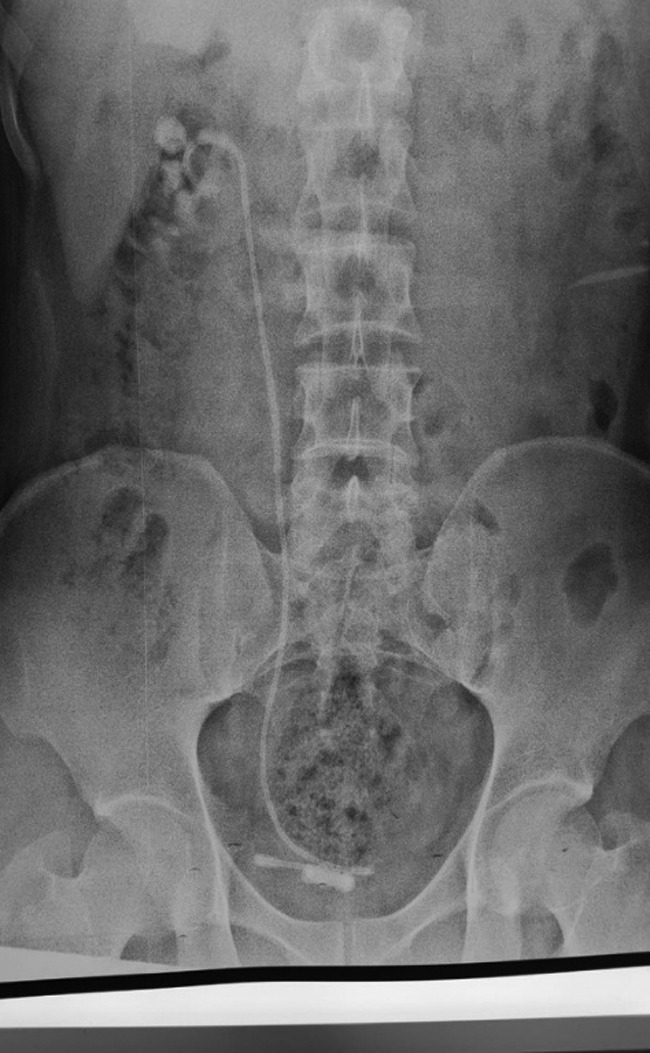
Plain KUB showed forgotten Double-J with the stone formation at both sides of the coils. KUB, kidney, ureter, and bladder radiograph.
